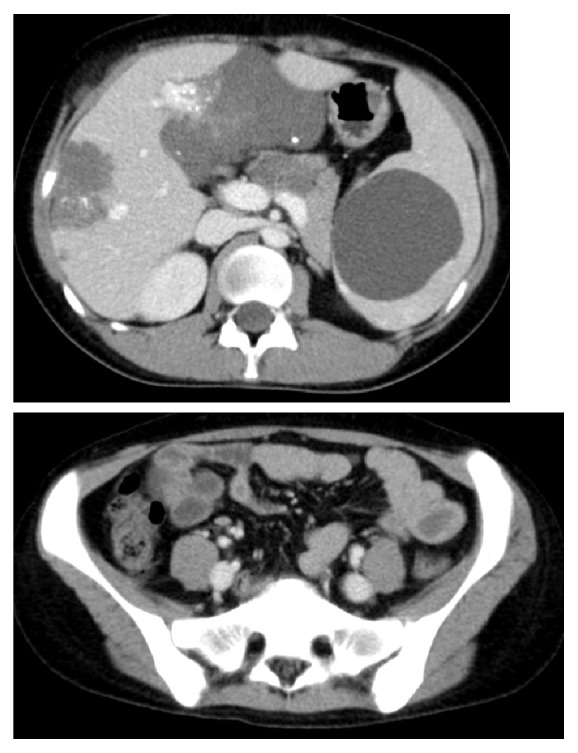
Computed tomography at 19 years of age revealed multiple VMs on almost the entire GI tract, liver, and spleen.
